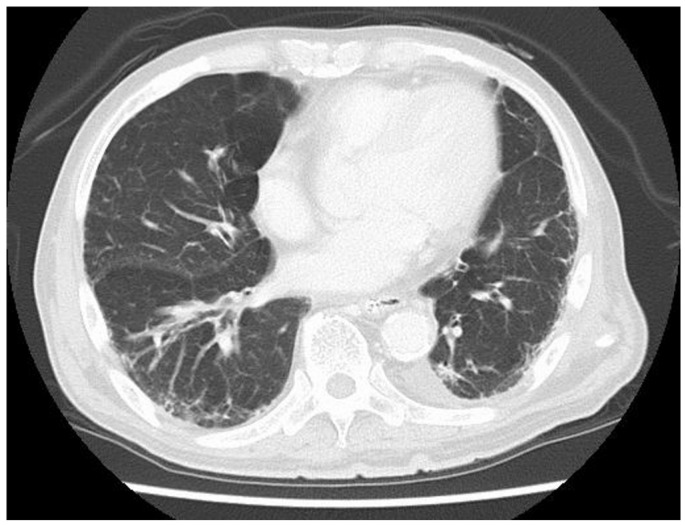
Thoracic CT showed mild aspiration pneumonia without exacerbation of interstitial pneumonia.
